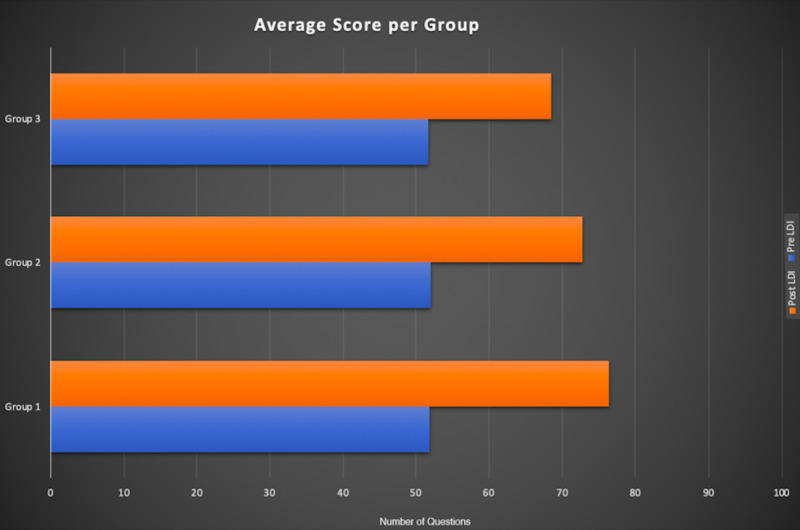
Breakdown of pre-/post-LDI assessments among different healthcare providers LDI, Laser Doppler Imaging
